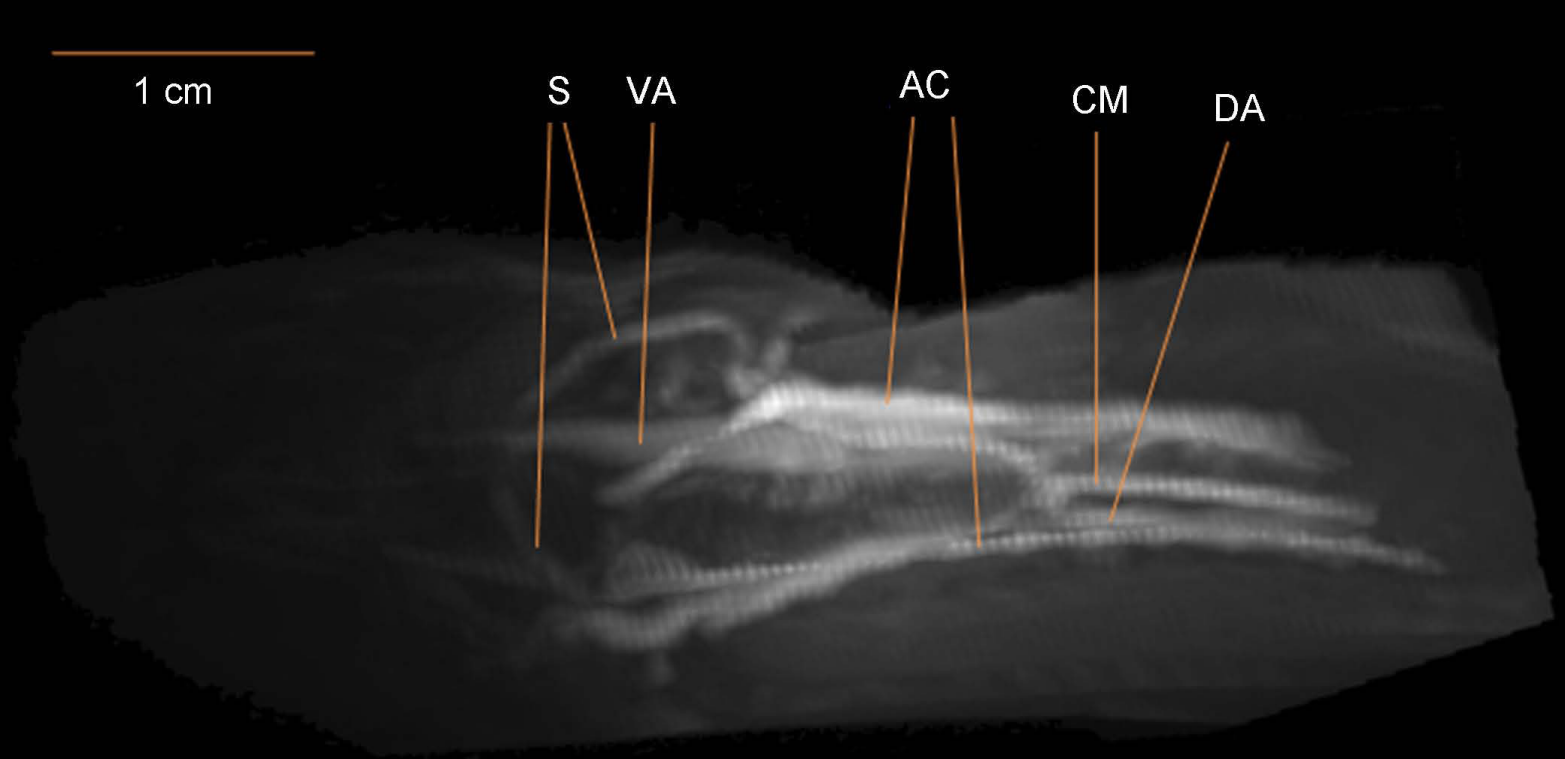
Dorsal view of the region 3-6 cm caudal to the snout of *Monopterus albus *perfused with contrast mix 2, with anterior to the left. The image was created by 3D-rendering software following MRI. Key: VA, ventral aorta; DA, dorsal aorta; S, shunt vessels joining ventral and dorsal aorta; CM, celiacomesenteric artery; AC, anterior cardinal veins.
